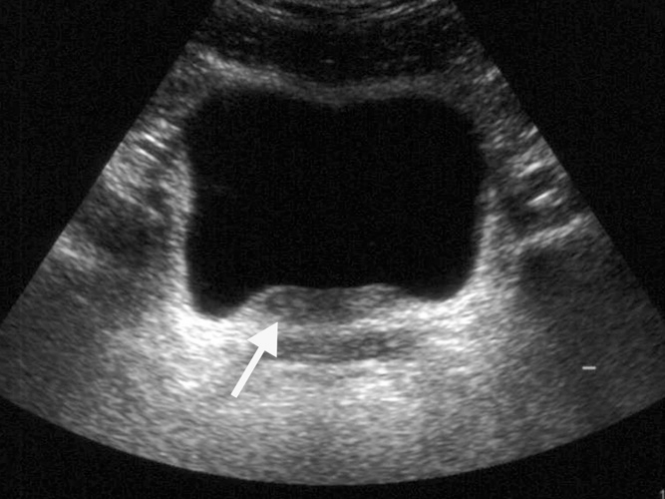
Ultrasonography shows thickening of the wall of the urinary bladder.
